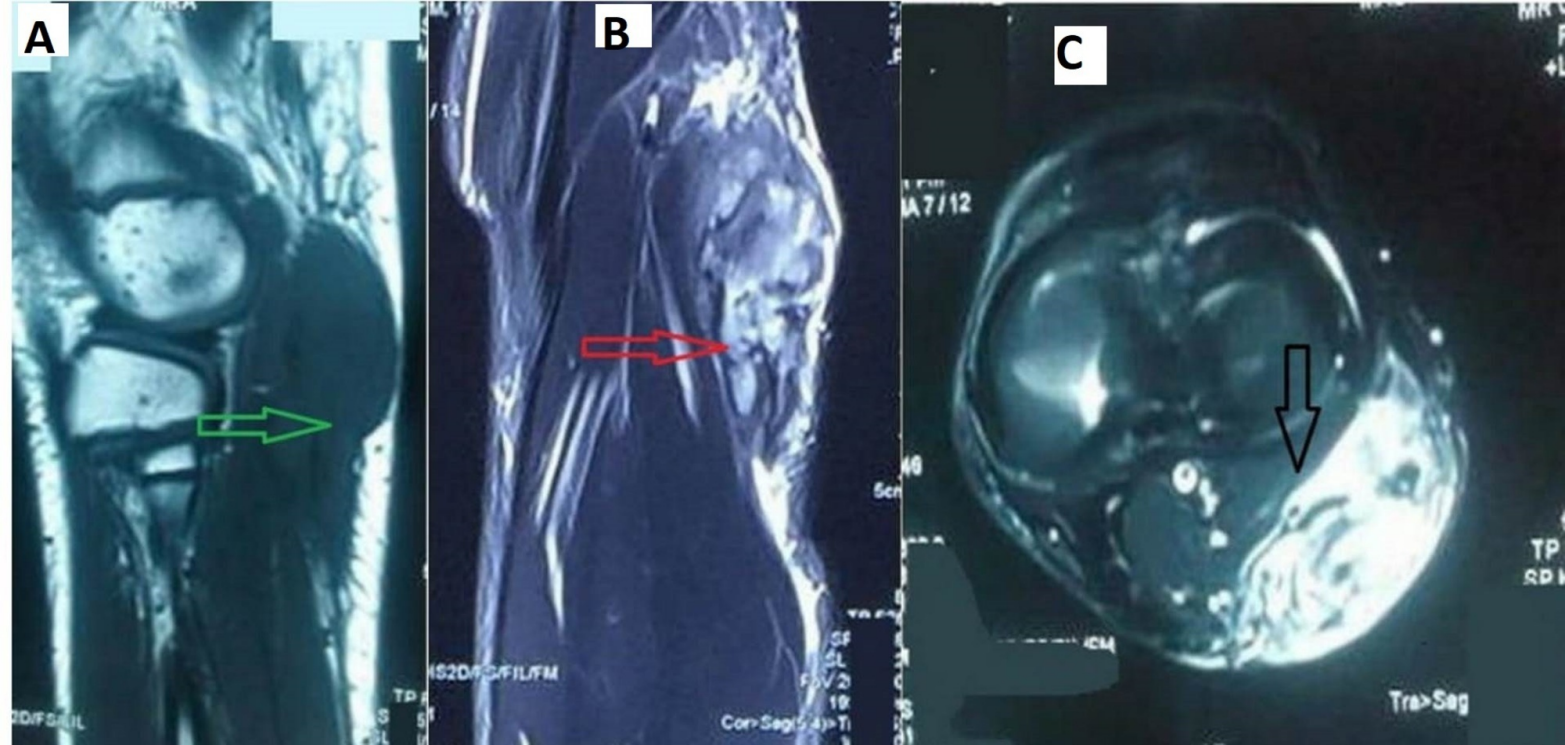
Magnetic resonance imaging showing an ill-defined, lobulated mass that is A) hyperintense on T2-weighted imaging (green arrow), B) hypointense on T1-weighted (red arrow) and C) STIR sequence with contrast enhancement adjacent to the lateral head of the gastrocnemius (black arrow). STIR: Short-TI Inversion Recovery.
